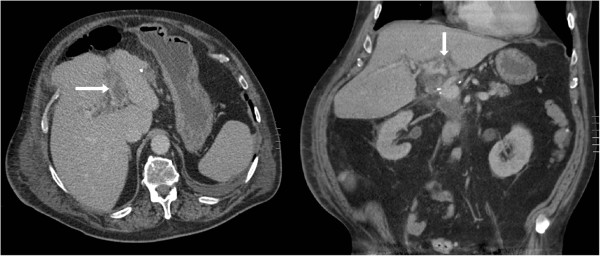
Postoperative CT abdomen with contrast showing left portal venous thrombosis (arrows) on axial and sagittal views.
